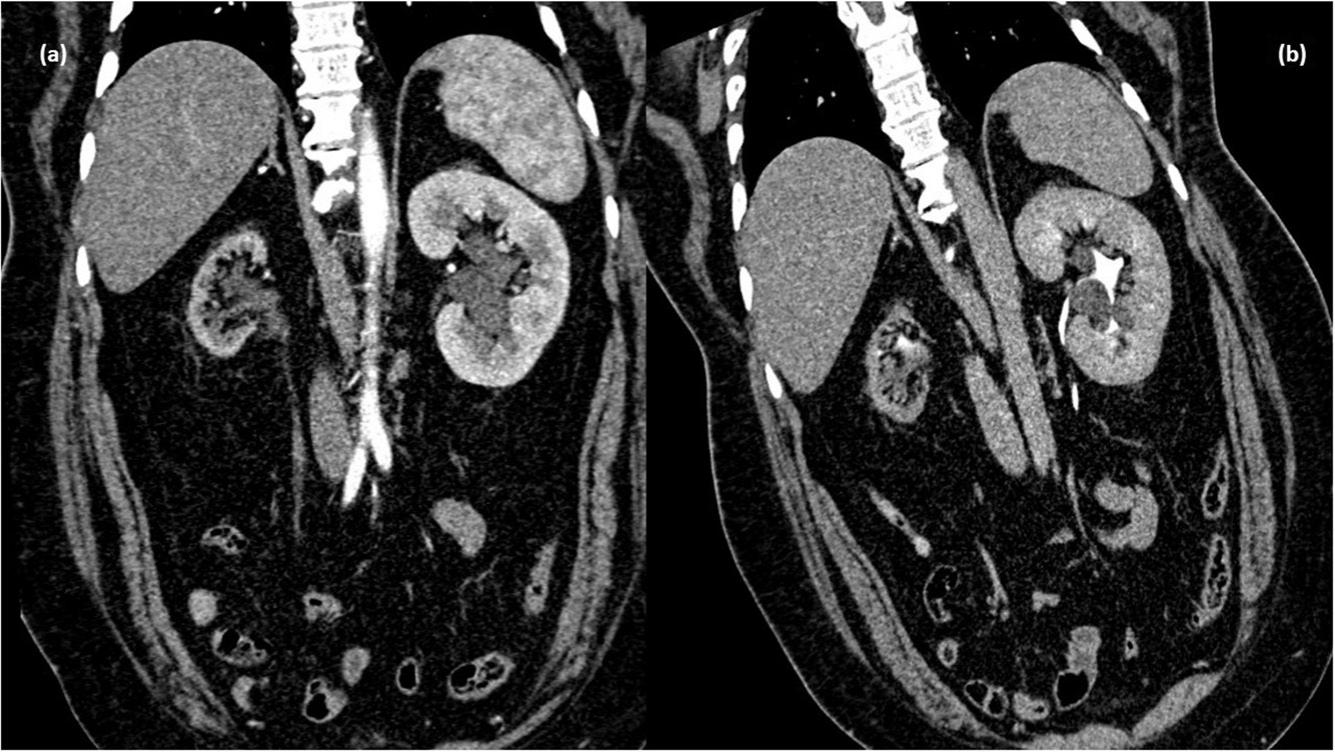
CT scan arterial phase in coronal plane showing non enhancing collection in peripelvic region of left kidney (d) MPR reformation of coronal plane of delayed phase of CT scan abdomen showing normal excretion of contrast with no evidence of leakage in PC system – unilateral peripelvic lymphangiectasia.
